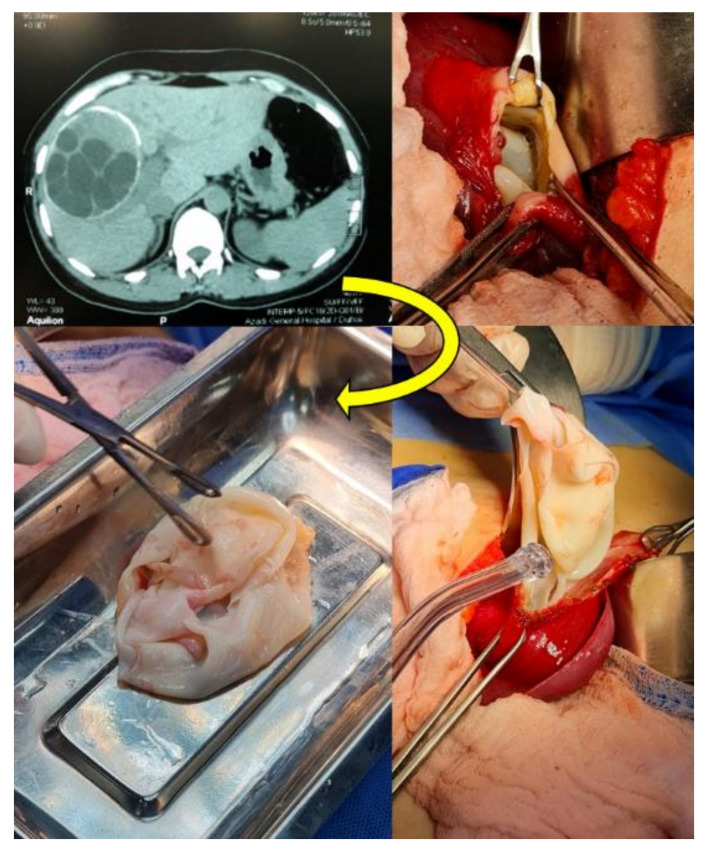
Computed tomography (CT) and the open surgery procedure for the excision of *Echinococcus granulosus sensu lato* cysts (CE3b stage) from a human liver in Duhok city, Duhok governatorate, Kurdistan region, Iraq.
